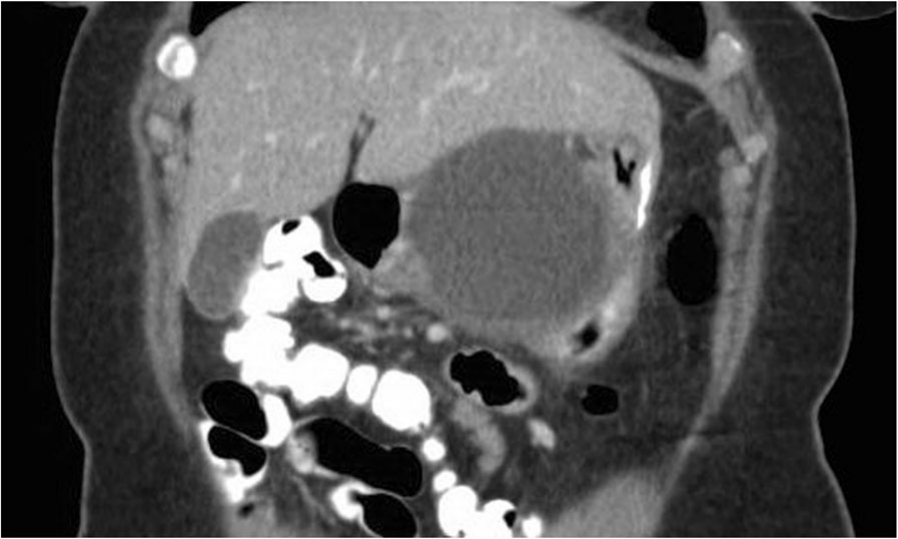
Sleeve compression (coronal CT image)
